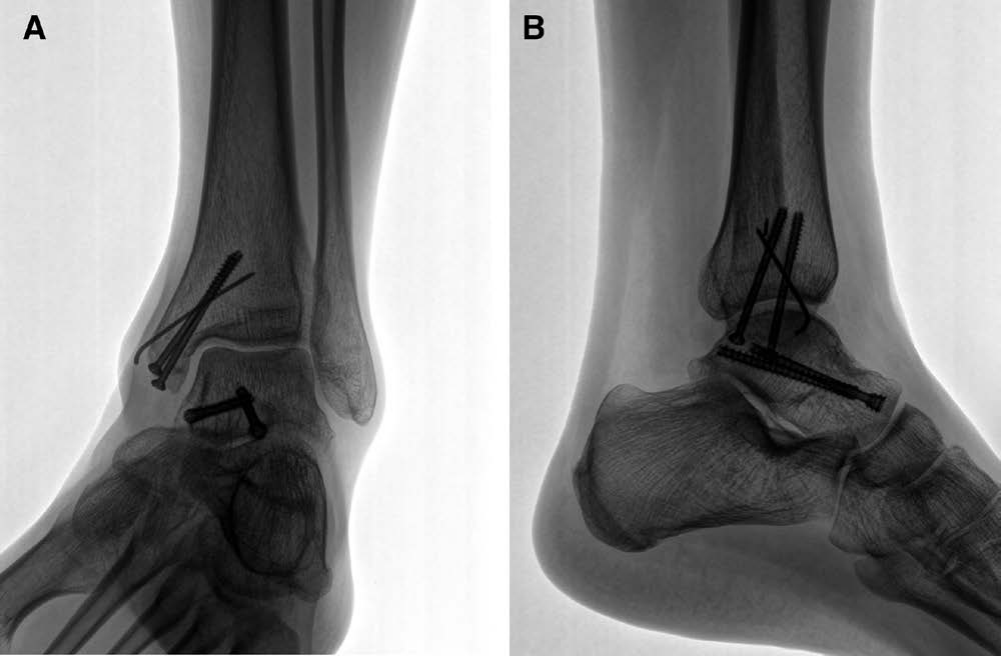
Postoperative follow-up radiographs showing fracture union. (A) Anteroposterior view revealing complete union of the talar neck and medial malleolar fractures. The presence of the Hawkins sign and absence of subchondral lucency indicate no evidence of avascular necrosis. (B) Lateral view confirming well-maintained ankle joint space without signs of early arthrosis..
